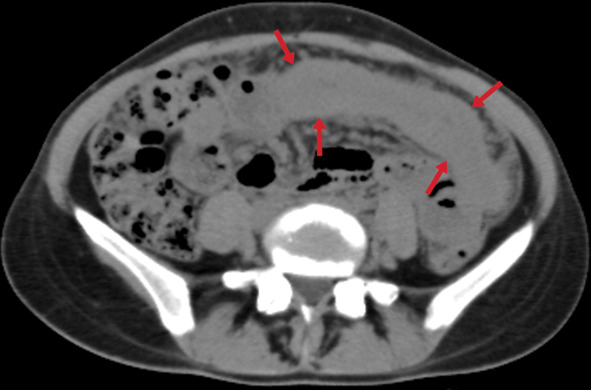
Transverse CT image without intravenous contrast of a 24-year-old female. Diffuse-symmetric wall thickening of the ileal segment is noted (arrows). Ileal TB
